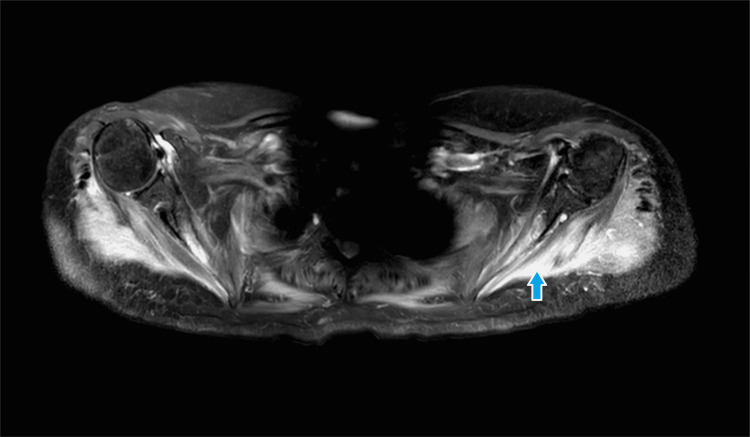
Magnetic resonance imaging (T2-weighted image) of shoulder joints showing high signals in shoulder girdle muscles.
